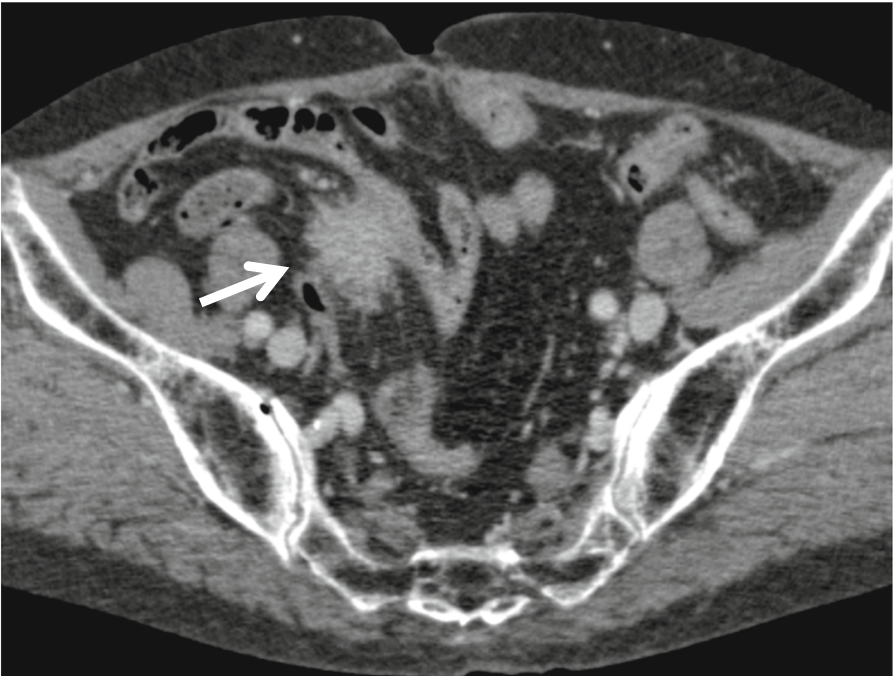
Abdominal enhanced CT, showing a radial, irregularly shaped mass (*white arrow*), 26 mm in diameter, at the root of the mesentery in the right lower quadrant and located close to the ileum
